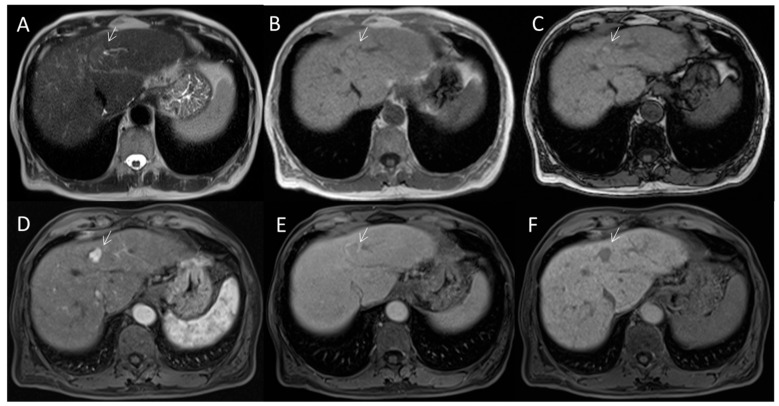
HCC on II seg. MRI assessment. The lesion (arrow) shows hyperintense signal on T2-W sequence (A), hypo-iso signal on T1 sequences ((B): in phase and (C): out phase) with APHE during arterial phase (D) of contrast study, capsule appearance during transitional phase (E) and hypointense signal in EOB phase (F).
